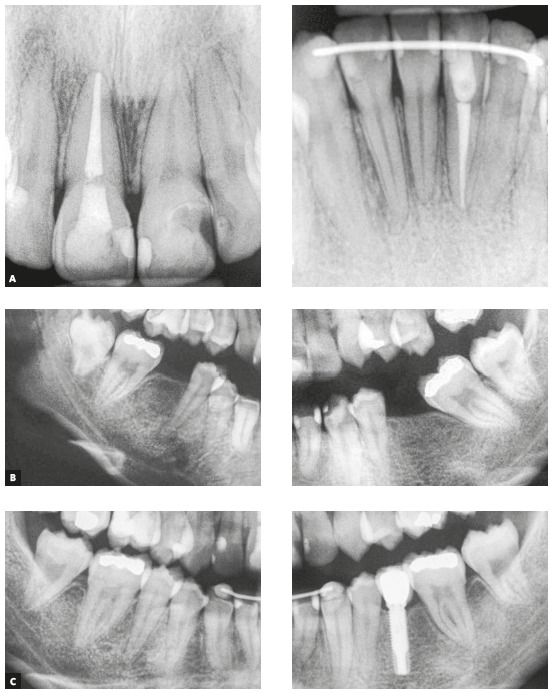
Final (A) periapical radiographs of maxillary and mandibular incisors, initial (B) periapical radiographs of the edentulous regions and final (C) periapical radiographs of the verticalized and mesialized mandibular second molars and the dental implant replacing tooth #35.
